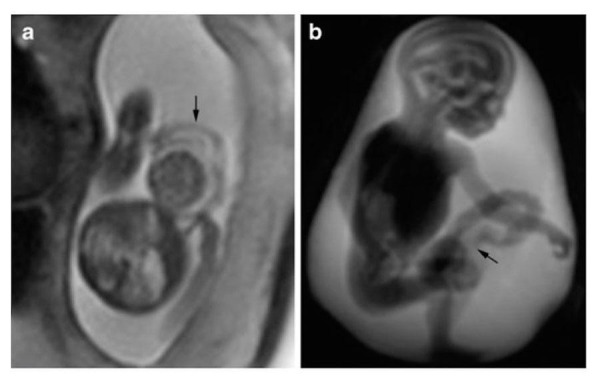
Omphalocele T2-weighted HASTE imaging of a 21-week gestational age fetus. Panel A is an axial image demonstrating intestinal loops lined with peritoneal membranes that are outside of the abdomen. Panel B is a sagittal slice demonstrating bowel loops outside of the abdomen as well as insertion of the umbilical cord. Image reprinted without change from Martin, et al. under the creative commons license.
